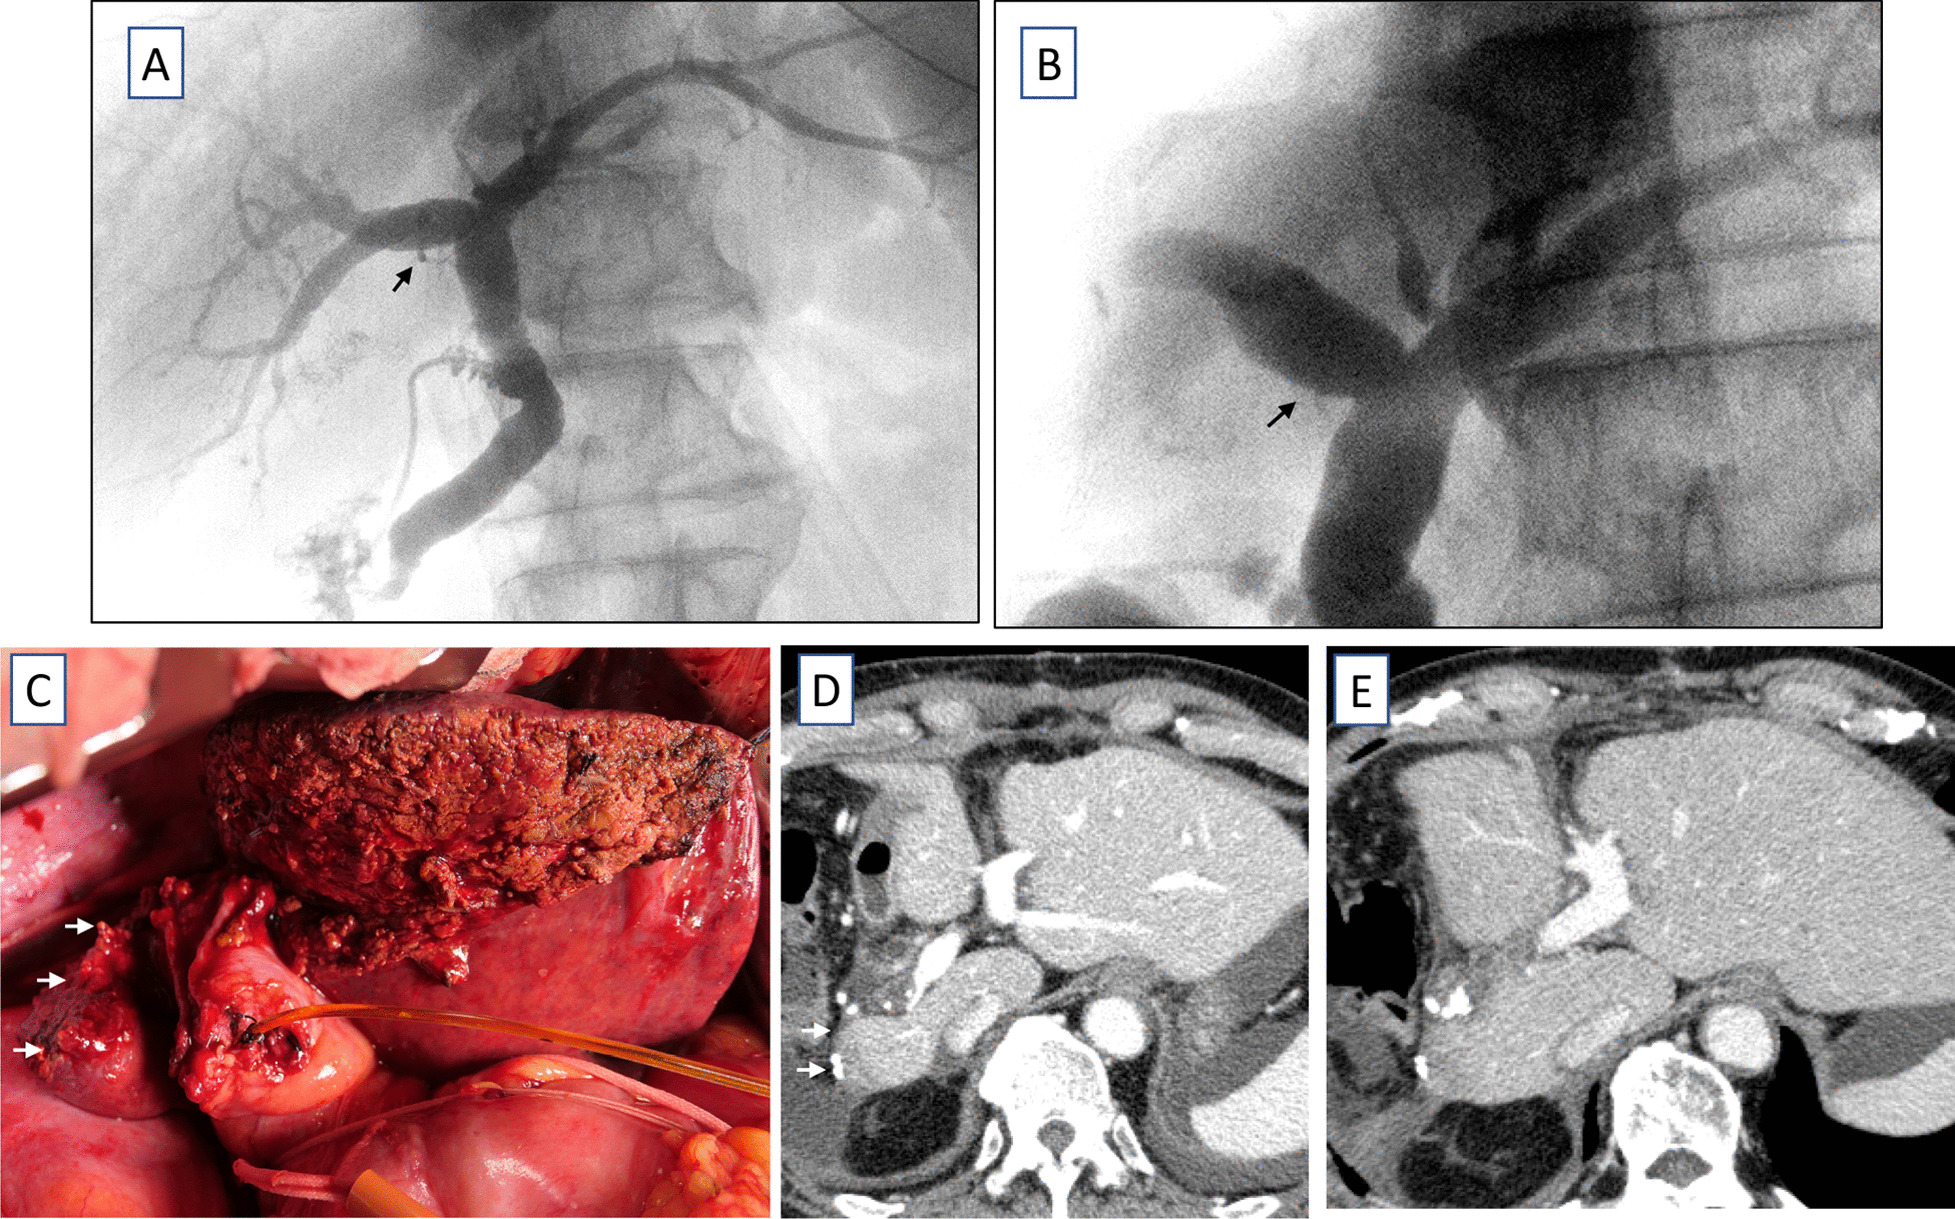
Intraoperative findings (A–C) and postoperative enhanced CT findings on postoperative day 5 and a month after surgery. A Intraoperative cholangiography showing the right caudate biliary branch. B Reevaluation of cholangiography after hepatic resection showing that the right caudate biliary branch could be preserved. C Intraoperative finding after hepatic resection preserved the right caudate lobe (white arrows). D, E Enhanced CT on postoperative day 7 and 1 month after surgery showing the preserved right caudate lobe (white arrows), *POD* postoperative day
